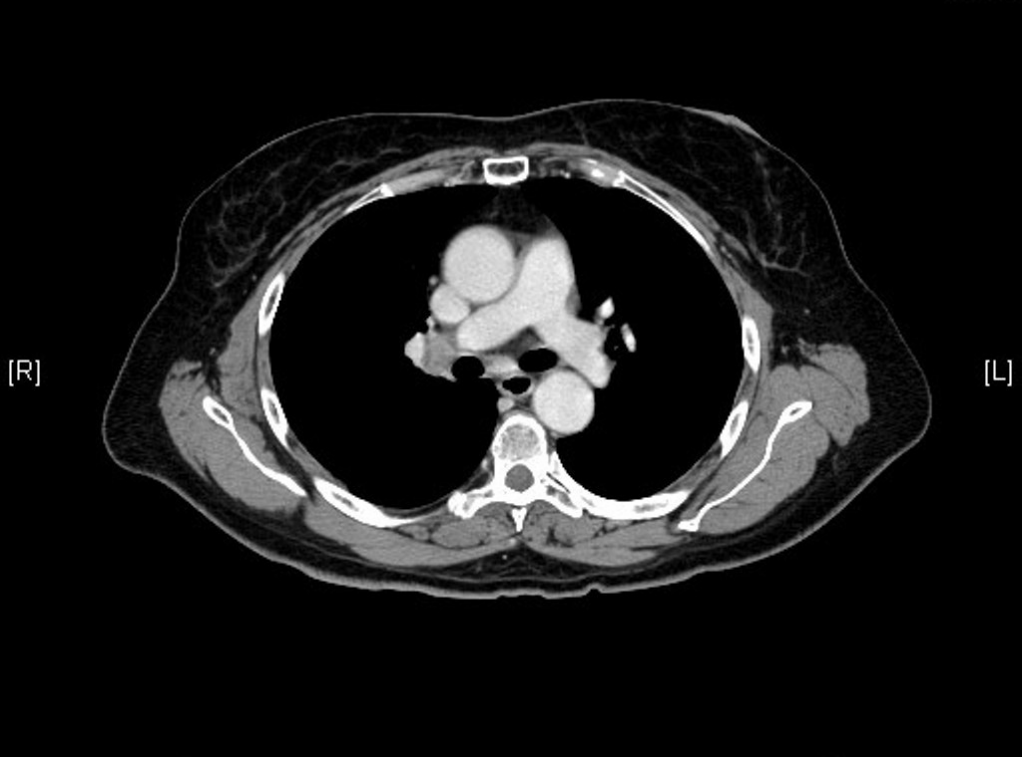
Hilar adenopathy seen on CT scan of the chest from 2004
